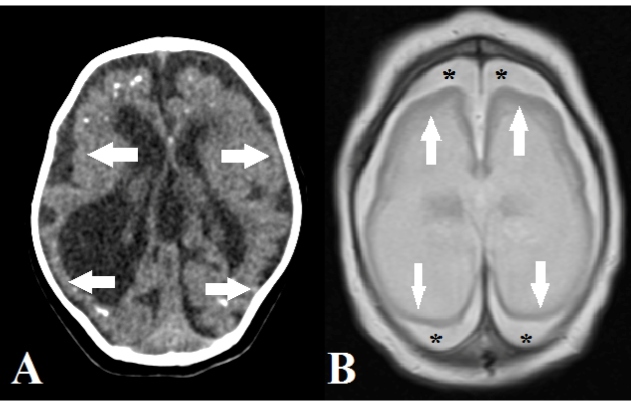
Axial computed tomography (A) and T2-weighted
